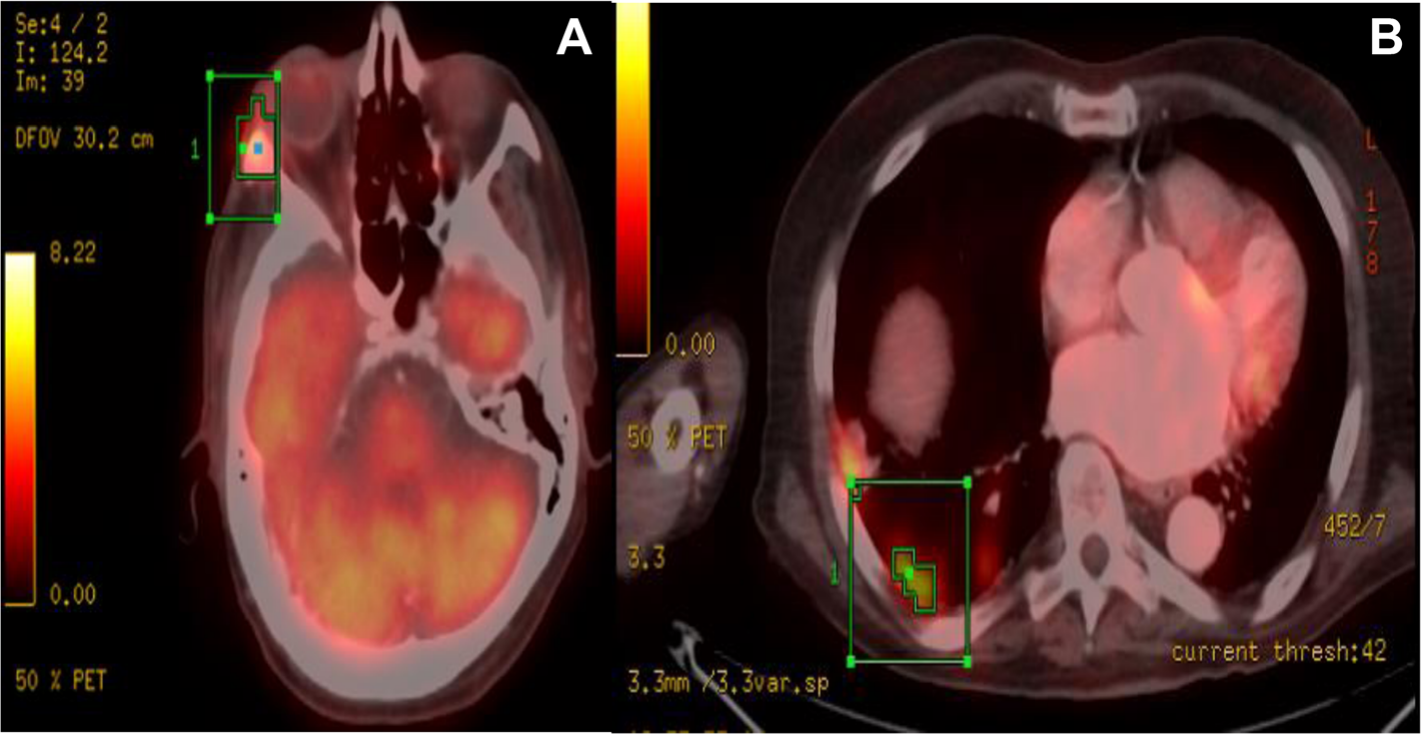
PET-CT Contrast-enhanced helical CT was performed and PET emission data 1 hour following IV injection of 18.1 mCi of F-18 FDG. A: Shows a new focus of abnormal metabolic activity at the lateral wall the right orbit with underlying sclerosis. Maximal SUV value is 4.6. B: Shows area of dense consolidation with high metabolic activity suggestive of malignancy rather than an infection or inflammation. Additionally, multiple hot spots involving the R. parotid, mandibular angle, lateral wall the R. orbit, sternum, both humeri and femurs were detectable.
